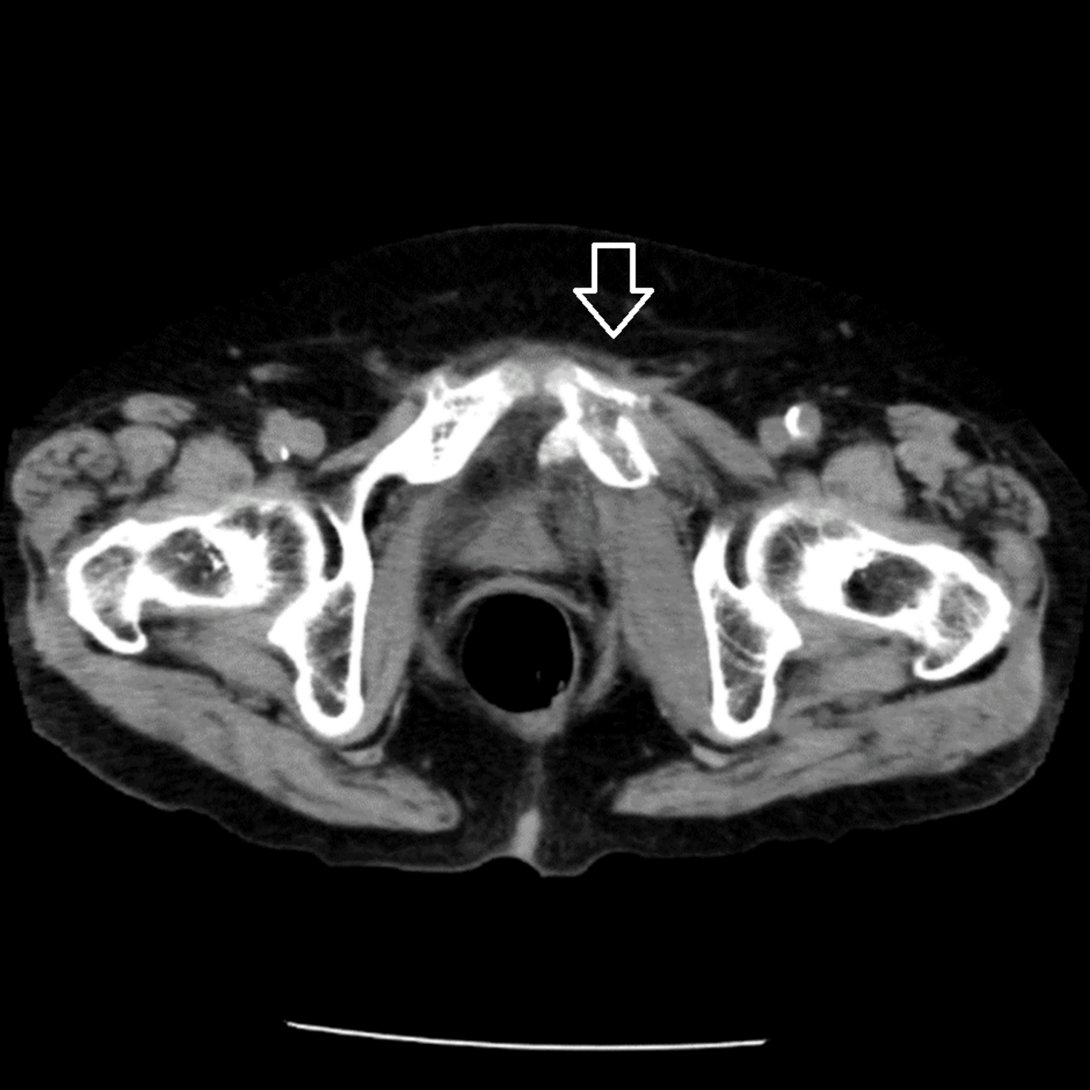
CT image immediately after the injury showing the tuberosity fracture. The arrow indicates a fracture of the left pubic bone and the resultant bone fragment.
